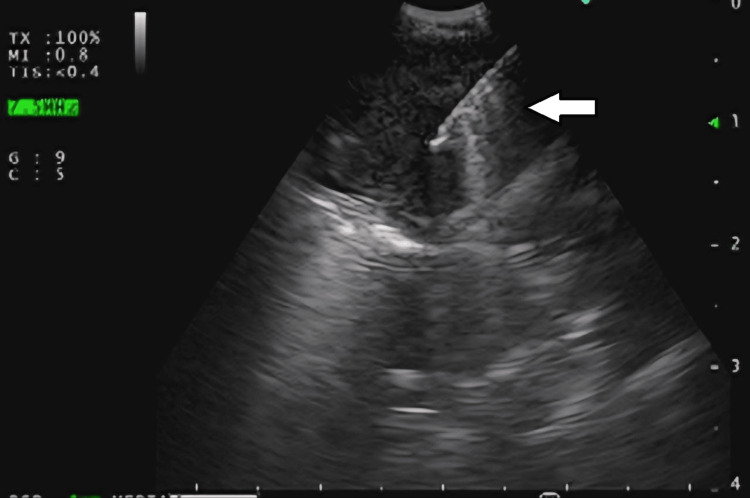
EBUS-TBNA of left interlobar lymph node. EBUS-TBNA: endobronchial ultrasound-guided transbronchial needle aspiration
